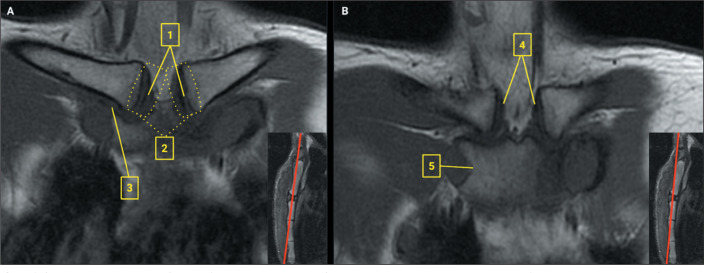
Sternal anatomy on MRI. A: Coronal T1-weighted image without fat
